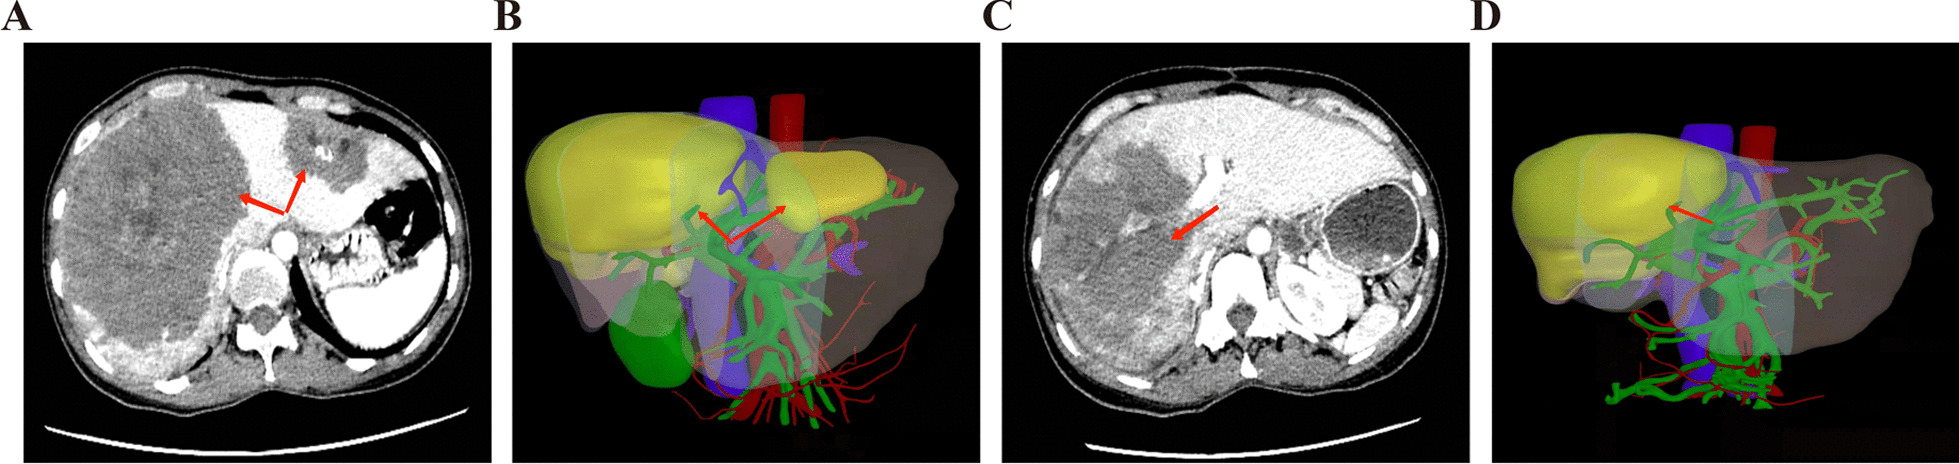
After TSH treatment, TSH2 patient, with FLRV/TLV > 30%, was able to receive ELRA treatment. A the first stage preoperative CT image; B the first stage preoperative digital 3D reconstruction of liver (FLRV/TLV < 30%); C CT image before the second stage operation; D the second stage preoperative digital 3D reconstruction of liver (FLRV/TLV > 30%)
